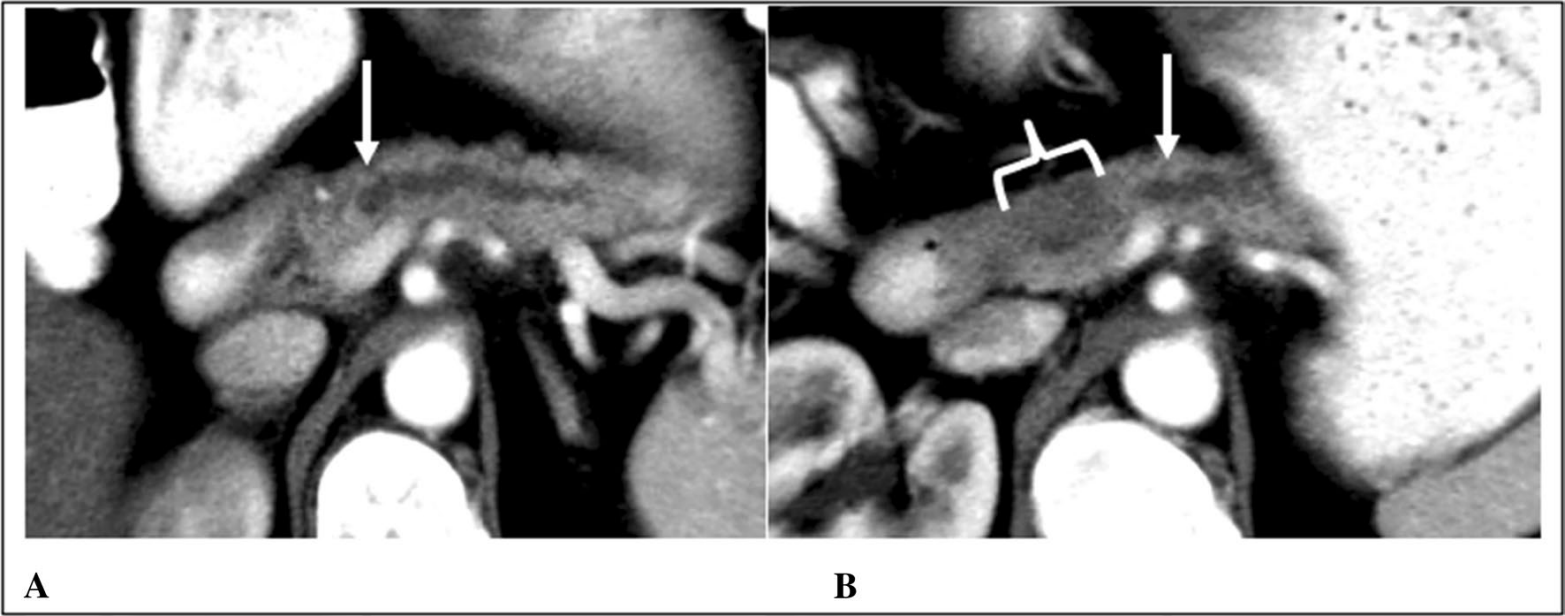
RADPEER 3 Score on CT—underread and missed findings. A case of a 48-year old female with epigastric pain. Axial postcontrast venous phase CT images (A, B) demonstrate pancreatic duct dilation (arrow) with abrupt termination in the head at a 20 mm hypodense mass (bracket). An example of an underread and missed finding with only a “prominent” pancreatic duct described in the initial interpretation. Both expert readers independently appreciated this mass with high confidence, with a RADPEER 3 score. The patient was eventually diagnosed with PDAC 6 months later, when she presented with recurrent pain, jaundice and weight loss and abdominal imaging revealed a pancreatic head mass
